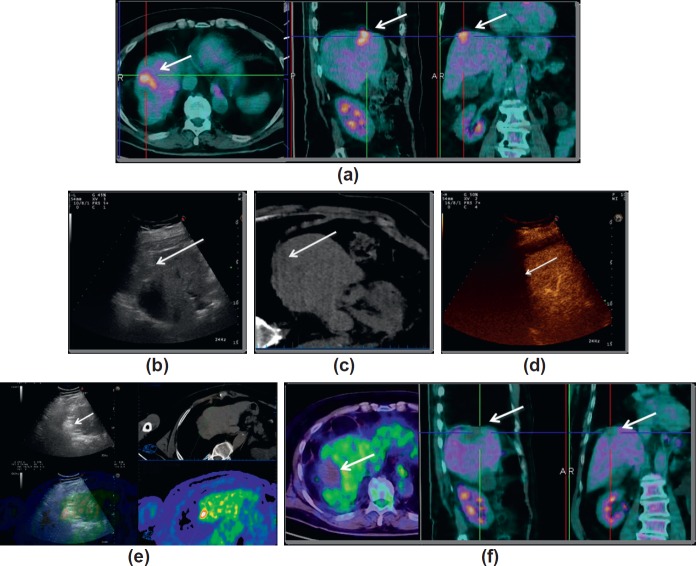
Case of a patient previously treated for colorectal cancer presenting with PET-positive metastasis that was treated with percutaneous microwave ablation guided by fusion imaging and virtual navigation. (a). PET/CT images demonstrating a 4 cm FDG avid recurrence (arrow) located at the liver dome. (b). The lesion was completely invisible at ultrasound (arrow). (c). The lesion was undetectable at non-enhanced CT too (arrow). (d). Even contrast-enhanced ultrasound was not able to show the lesion due to the presence of interposed aerated lung parenchyma (arrow). (e). The treatment was performed using a system for image fusion that shows the real-time ultrasound in the upper left quadrant (arrow gas forming during ablation), the CT images on the upper right quadrant, the PET images in the lower right quadrant and the fused images in the lower left quadrant. (f). PET/CT after treatment showing complete ablation of the treated lesion.
